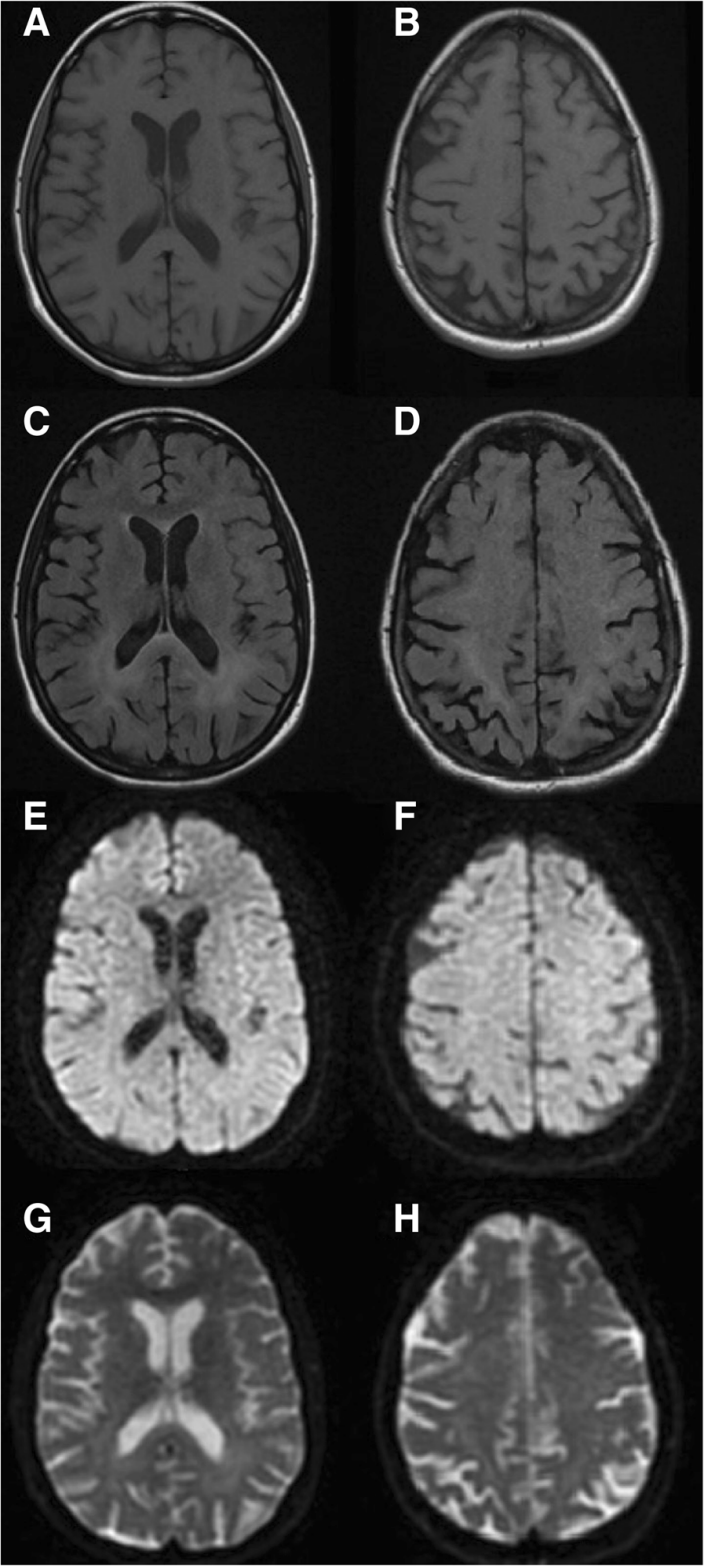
Brain MRI. Axial T1 (a-b), T2/FLAIR (c-d), DWI (e-f) and ADC-map (g-h). Bilateral relatively symmetric periventricular and deep white matter T2/FLAIR hyperintensity predominantly in the posterior supratentorial area. Areas of restricted diffusion were not observed
